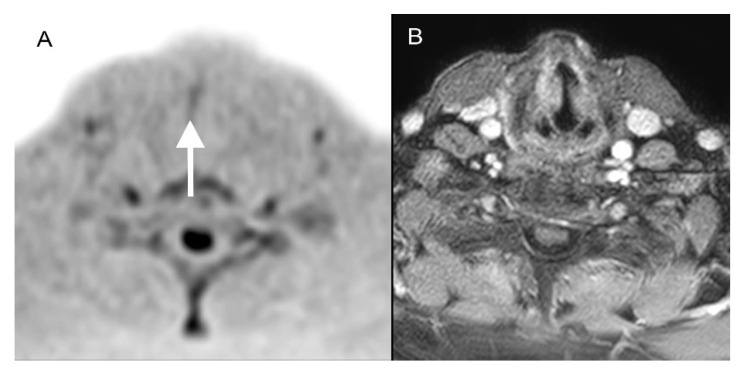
An 80-year-old patient with a pT1a cN0 cM0 squamous cell carcinoma of the vocal cord on the right side, which was diagnosed correctly in DWIBS ((A); white arrow) by both readers but not in contrast-enhanced transversal T1-weighted scans with fat saturation (B).
